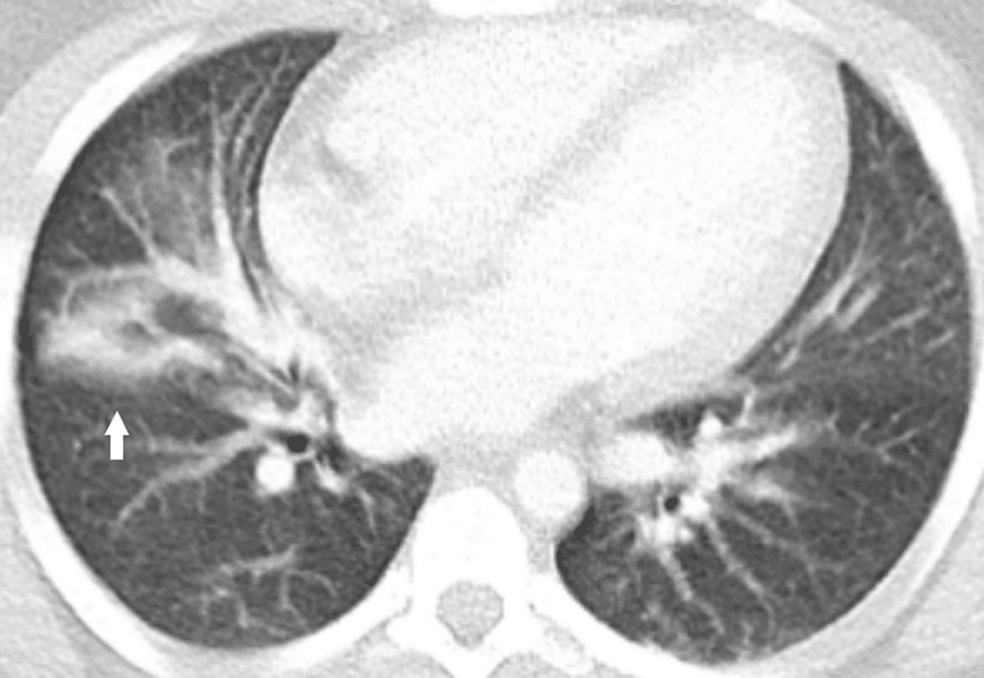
Chest CTA on admission showing a moderate linear consolidation in the right middle lobe (arrow) CTA: computed tomography angiography
